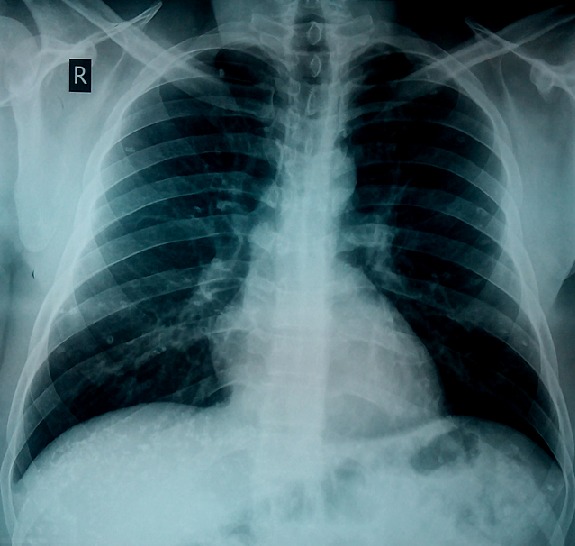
Posteroanterior chest radiograph showing bilateral, multiple, crescentic calcific opacities in both lungs and in the region of the liver
